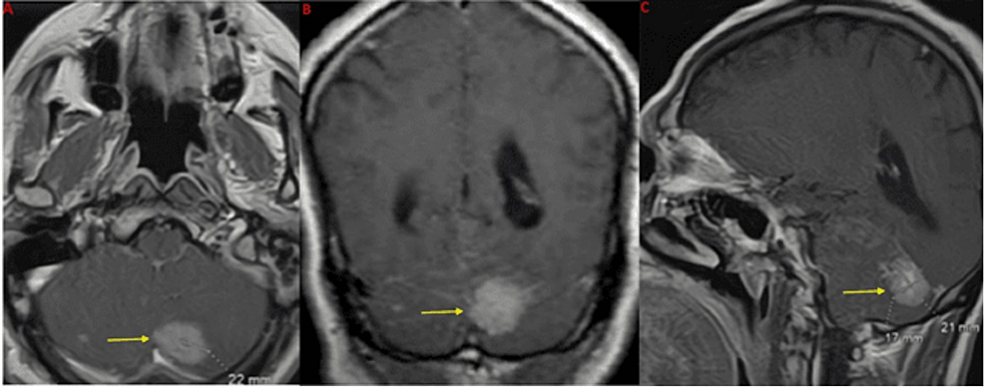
Preoperative MRI of the brain postcontrast sequences in the (A) axial, (B) coronal, and (C) sagittal planes, respectively Yellow arrows point to the postcontrast-enhancing mass MRI: magnetic resonance imaging
